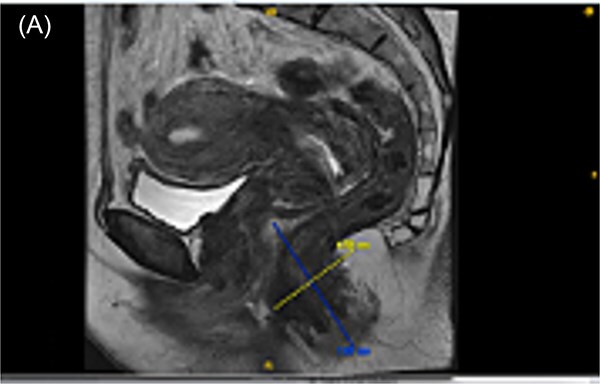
MR pelvis with and w/o contrast [MN2]. (A) MRI demonstrating T1 Hyperintense anal lesion with restricted diffusion and early contract enhancement.
